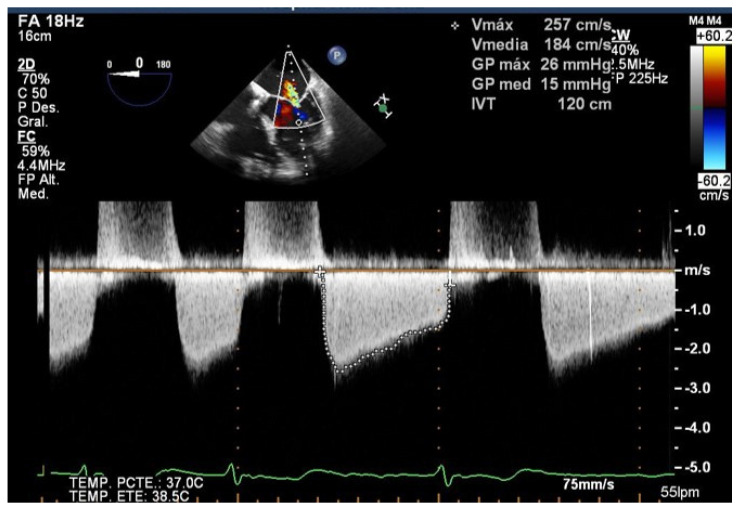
Severe mitral stenosis after implantation of a mitral clip measured by Doppler echo.
